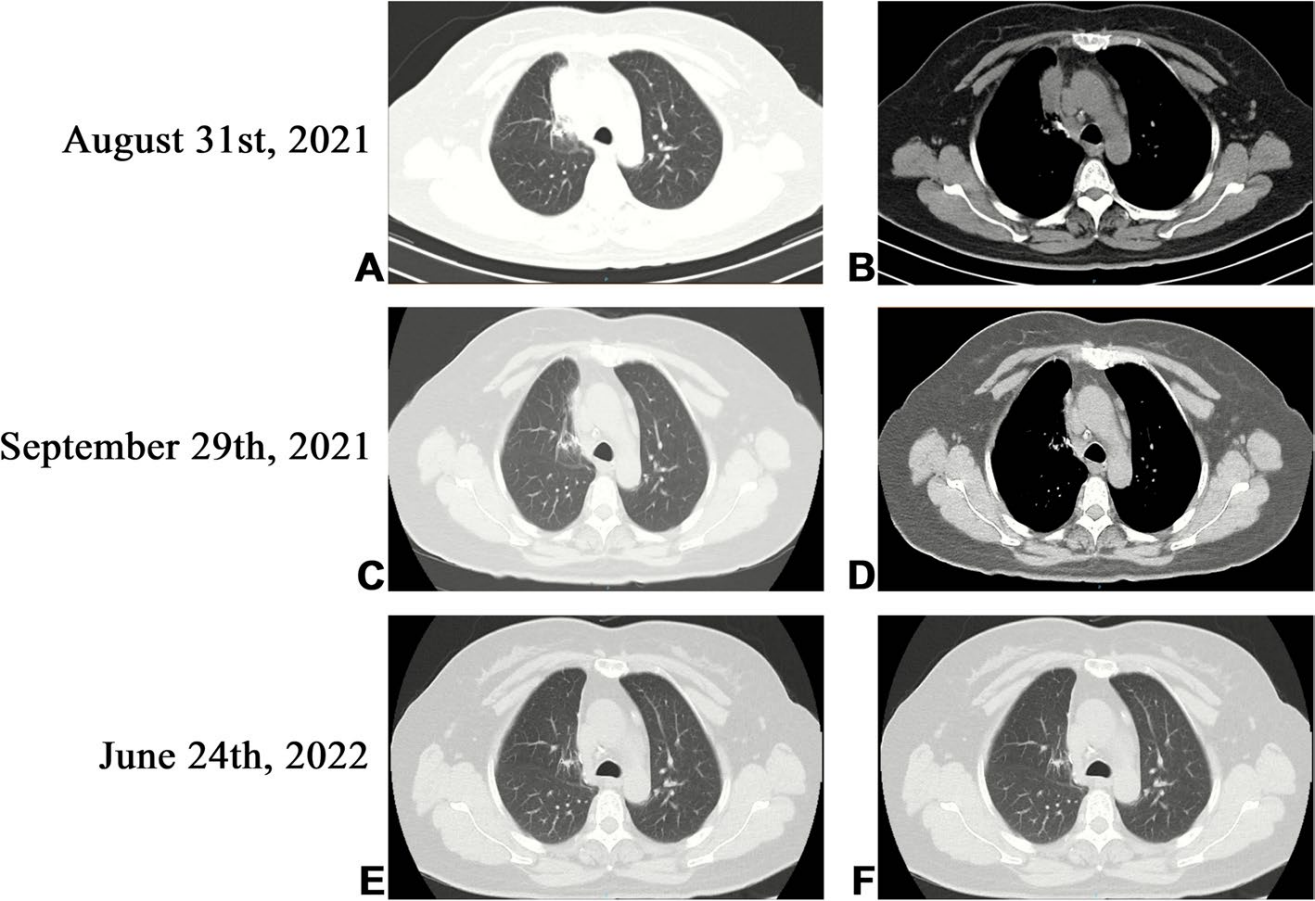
Postoperative reexamination of CT. August 31st, 2021. One month after surgery, HRCT showed the absence of the upper lobe of the right lung, the linear high-density shadow of the right hilar, and the adjacent patchy soft tissue shadow, considering the possibility of postoperative changes. There is a little effusion in the right interlobar fissure (A, B). September 29th, 2021. Two months after surgery, CT showed the absence of the upper lobe of the right lung, the linear high-density shadow of the right side of the lung, and the adjacent patchy soft tissue shadow, which was slightly smaller than that of 1 month after operation. There was a little effusion in the right interlobar fissure, which was slightly less than before (C, D). June 24th, 2022. Ten months after surgery, CT showed the absence of the upper lobe of the right lung, the linear high-density shadow in the right hilar area and the adjacent cord shadow, and the soft tissue shadow disappeared 2 months after operation (E, F)
